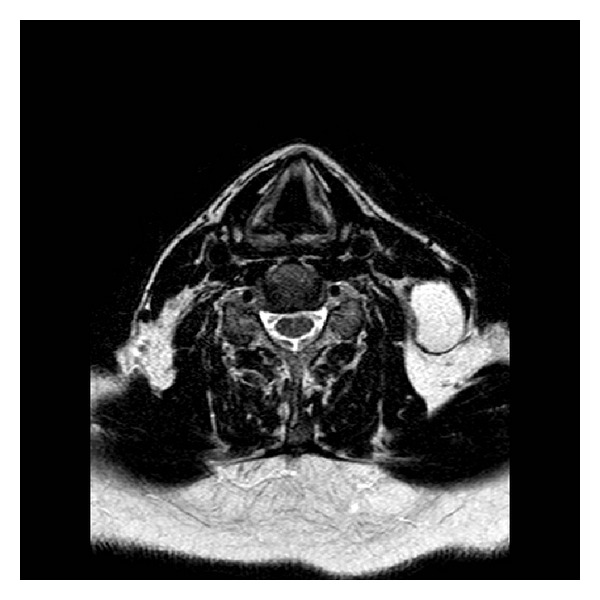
Axial slides of T2-weighted MRI showing a hyperintense cystic lesion behind the left sternocleidomastoid muscle in the neck level five.
